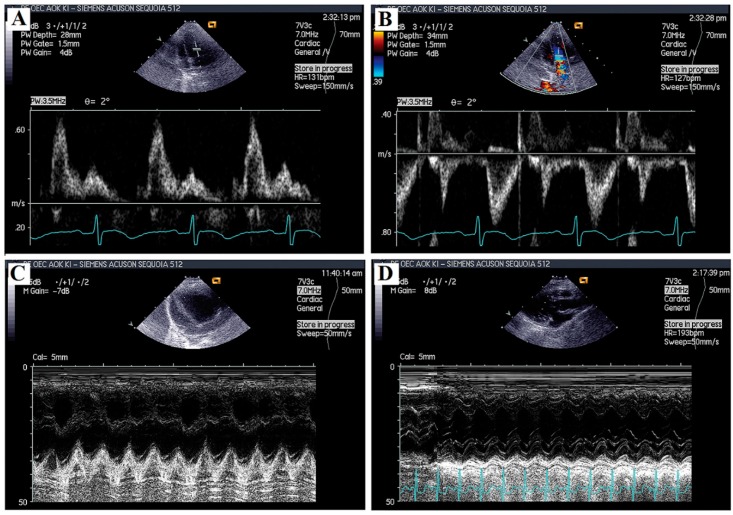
Representative images of echocardiographic measurements. (A) Doppler image, mitral inflow velocities; (B) color Doppler image, velocity of left ventricle outflow tract; (C) M-mode image, tricuspidal annular plane systolic excursion (TAPSE); (D) M-mode image, parasternal long axis view (PLAX) of left ventricle.
